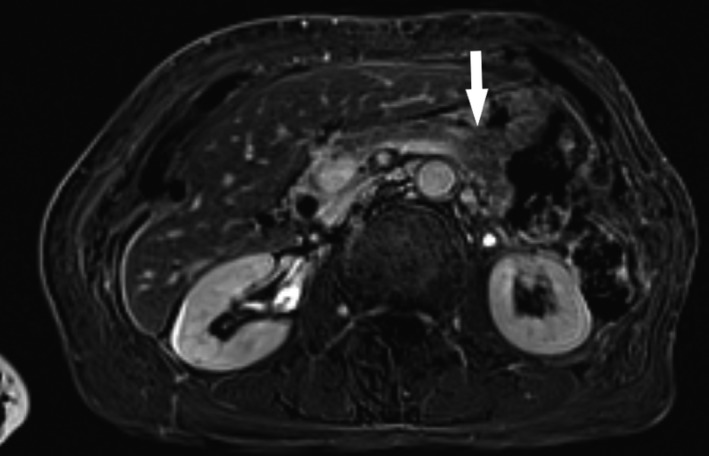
MRI revealing a 2.5‐cm pancreatic LEC at the body of the pancreas
